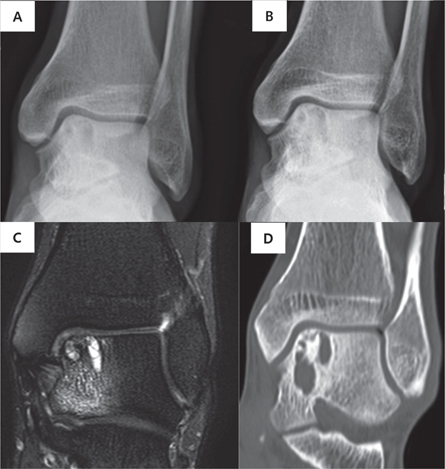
A 48-year-old man with OCL. (A) Initial standing radiograph and (C) MRI. (B) Standing radiograph and (D) CT at 3.8-year follow-up. Although the lesion size increased, the AOFAS ankle–hindfoot score improved from 71 to 80.
